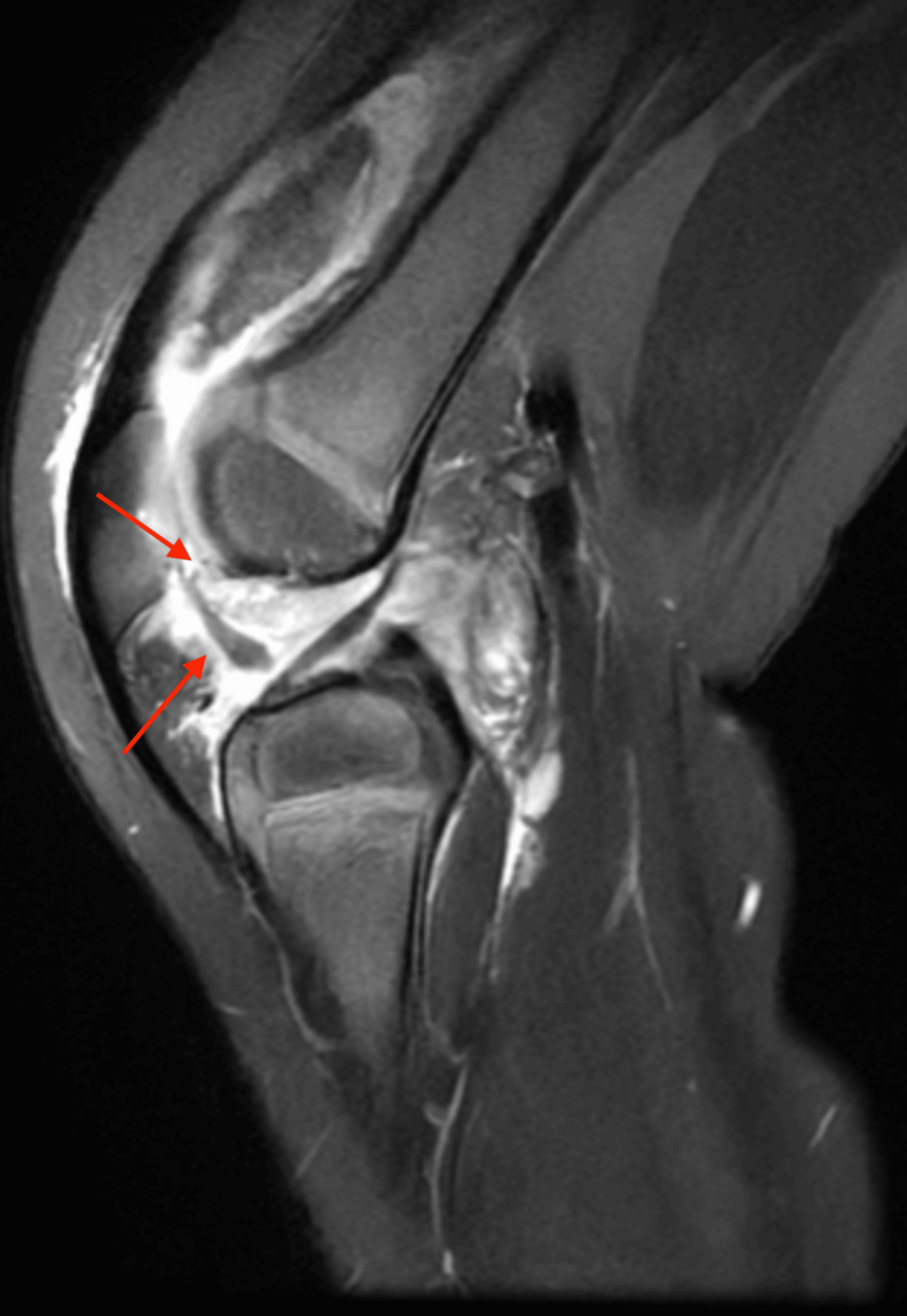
Lateral Magnetic Resonance Imaging of the Right Knee Showing Synovial Thickening and Irregularity Involving the Suprapatellar Recess, Patellofemoral Area, Infrapatellar Fat Pad, Anterior and Posterior Cruciate Ligaments, and Posterior Condyles (Red Arrows)
